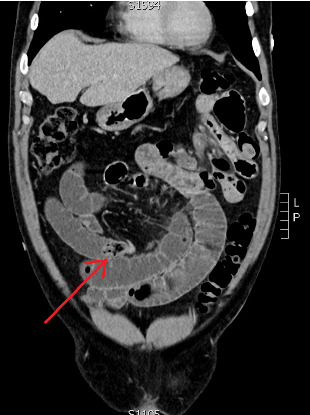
CT coronal view with the arrow marking the transition point of the small bowel obstruction with the enterolith visible just proximal to the collapsed loop of small bowel distally.
